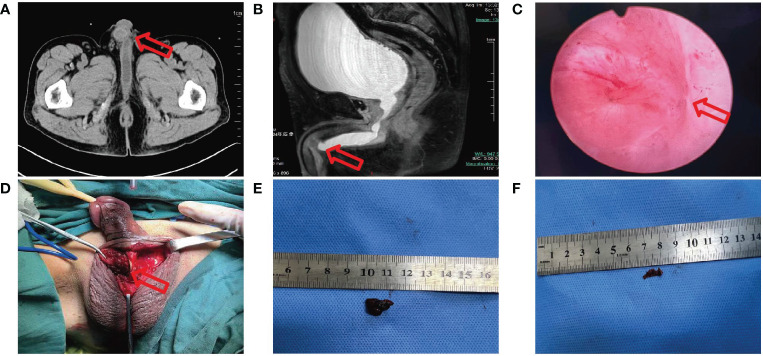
(A) CT image shows a mass measure 1.1 cm×1.4 cm in the middle cavernous body of penile with proximal urethral dilation. (B) MRI image shows a mass measure 1.2 cm×1.3 cm in the middle cavernous body of penile with local urethral stricture. (C) Electronic cystoscope image shows urethra was crushed. (D-F) During operation a fused nodule was found on the right side of the corpus cavernosum.
